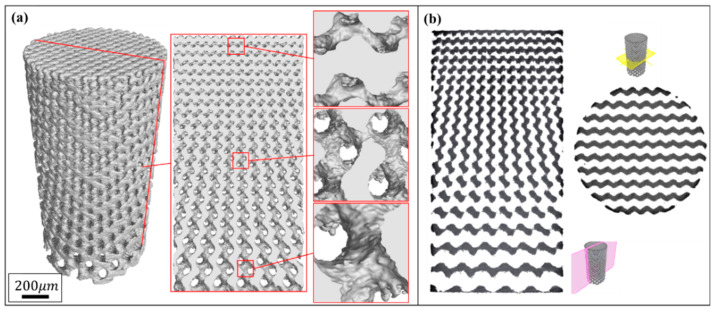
(a) Micro-computer tomography CT) reconstruction models of Gyroid cell-size gradient samples with different magnifications, and (b) corresponding 2D slice images.
